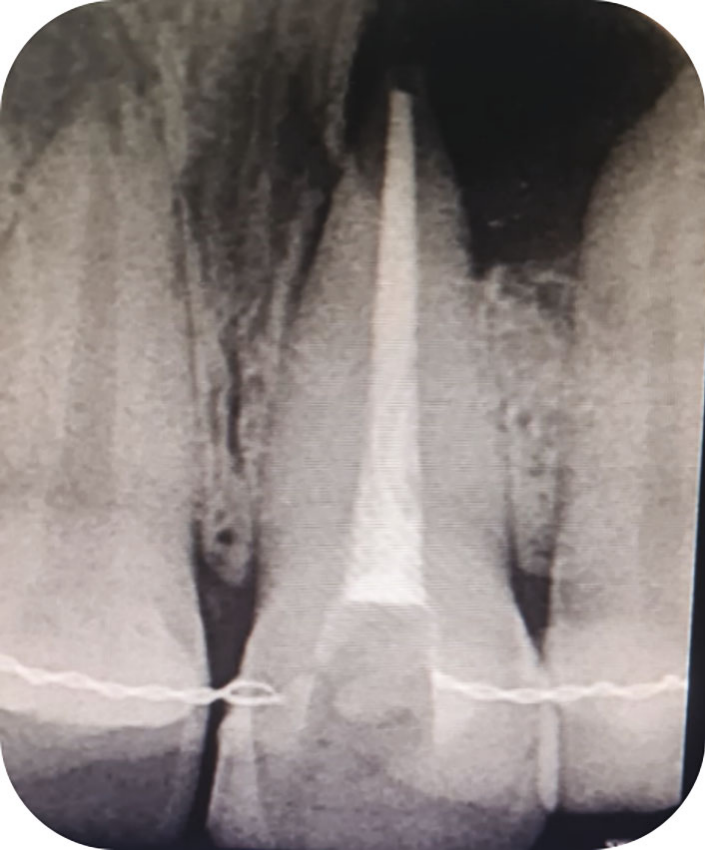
Radiographic showed root canal obturation after root canal retreatment.
